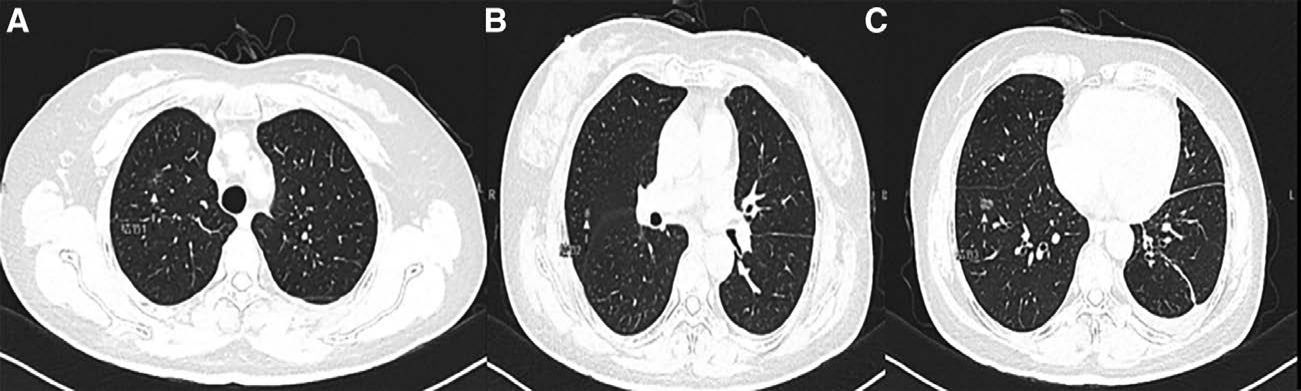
CT images before surgery. (A) Nodule 1 in the apical segment of the upper right leaflet. (B) Nodule 2 in the anterior segment of the right upper lobe. (C) Nodule 3 in the anterior basal segment of the right lower lobe. CT = computed tomography.
